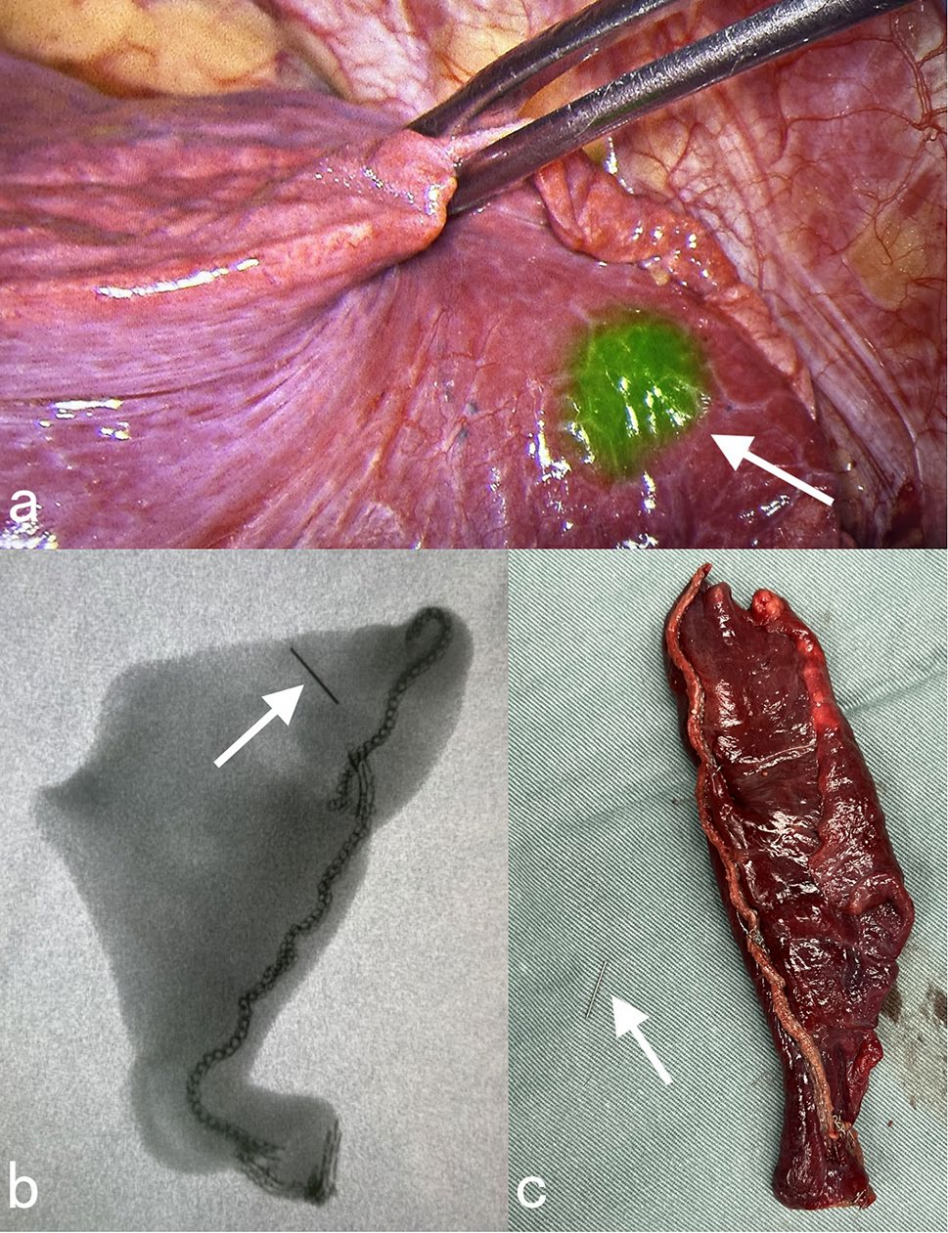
Intraoperative conditions: (a) indocyanine green localization area (white arrow); (b) X-ray perspective of resected lung tissue showed the location of the needle (white arrow); (c) removed needle (white arrow)
